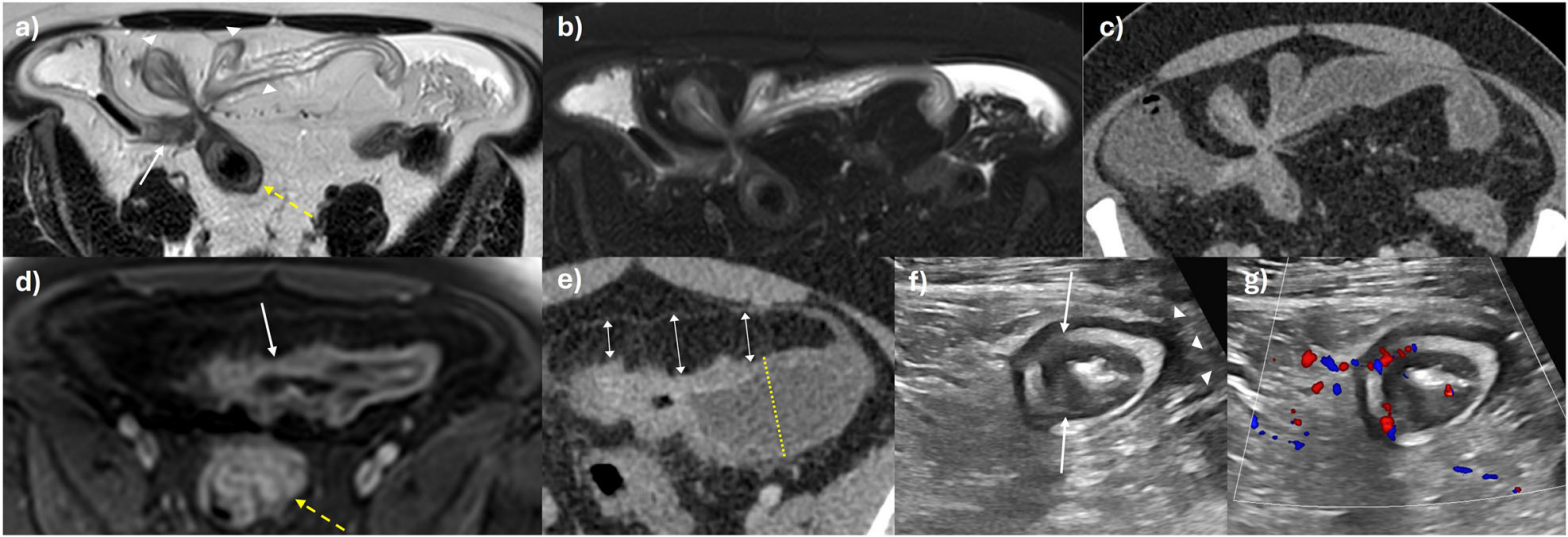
Active disease with penetrating and stricturing complications in a 36-year-old male patient with worsening symptoms, blood, and stool inflammatory markers. a Axial T2-weighted and (b) axial T2-weighted fat-suppressed images showing complex, fistulating disease of multiple terminal (arrow) and distal ileal loops (arrowheads), and also sigmoid colon (dashed arrow) in a ‘starfish’ configuration. There is severe activity with mural oedema matching the signal intensity of free fluid and perimural oedema, including free fluid. c Findings are shown on a CT study with intravenous contrast performed one day prior to MRE. d Axial post-contrast T1-weighted image shows severe active disease in the distal ileum upstream of the fistula (arrow) and also the distal sigmoid colon (dashed arrow) with layered transmural hyperenhancement. e Persistent narrowing of this same distal ileal segment on CT with mural hyperenhancement and new associated obstruction due to mixed stricturing, active disease (dashed line). There is mesenteric fat wrapping as a sign of chronic disease (arrows). f US performed one week later shows an active skip lesion in a mid-ileal loop with mural thickening, patchy hypoechoic submucosal changes, and loss of mural stratification (arrows). The mesenteric border is blurred (arrowheads). g There is a corresponding increased colour Doppler signal in these inflamed areas. Patchy mixed hyper- and hypoechoic changes in the mesentery indicated active disease on a background of chronic disease
